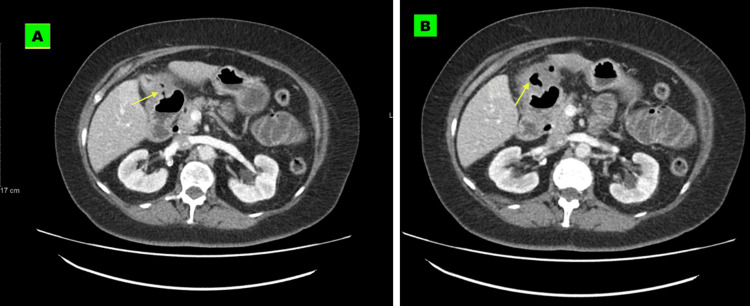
Axial CT slices at a lower level showing a track connecting the gallbladder with the duodenum consistent with cholecystodoudenal fistula (A and B) CT: Computed tomography
